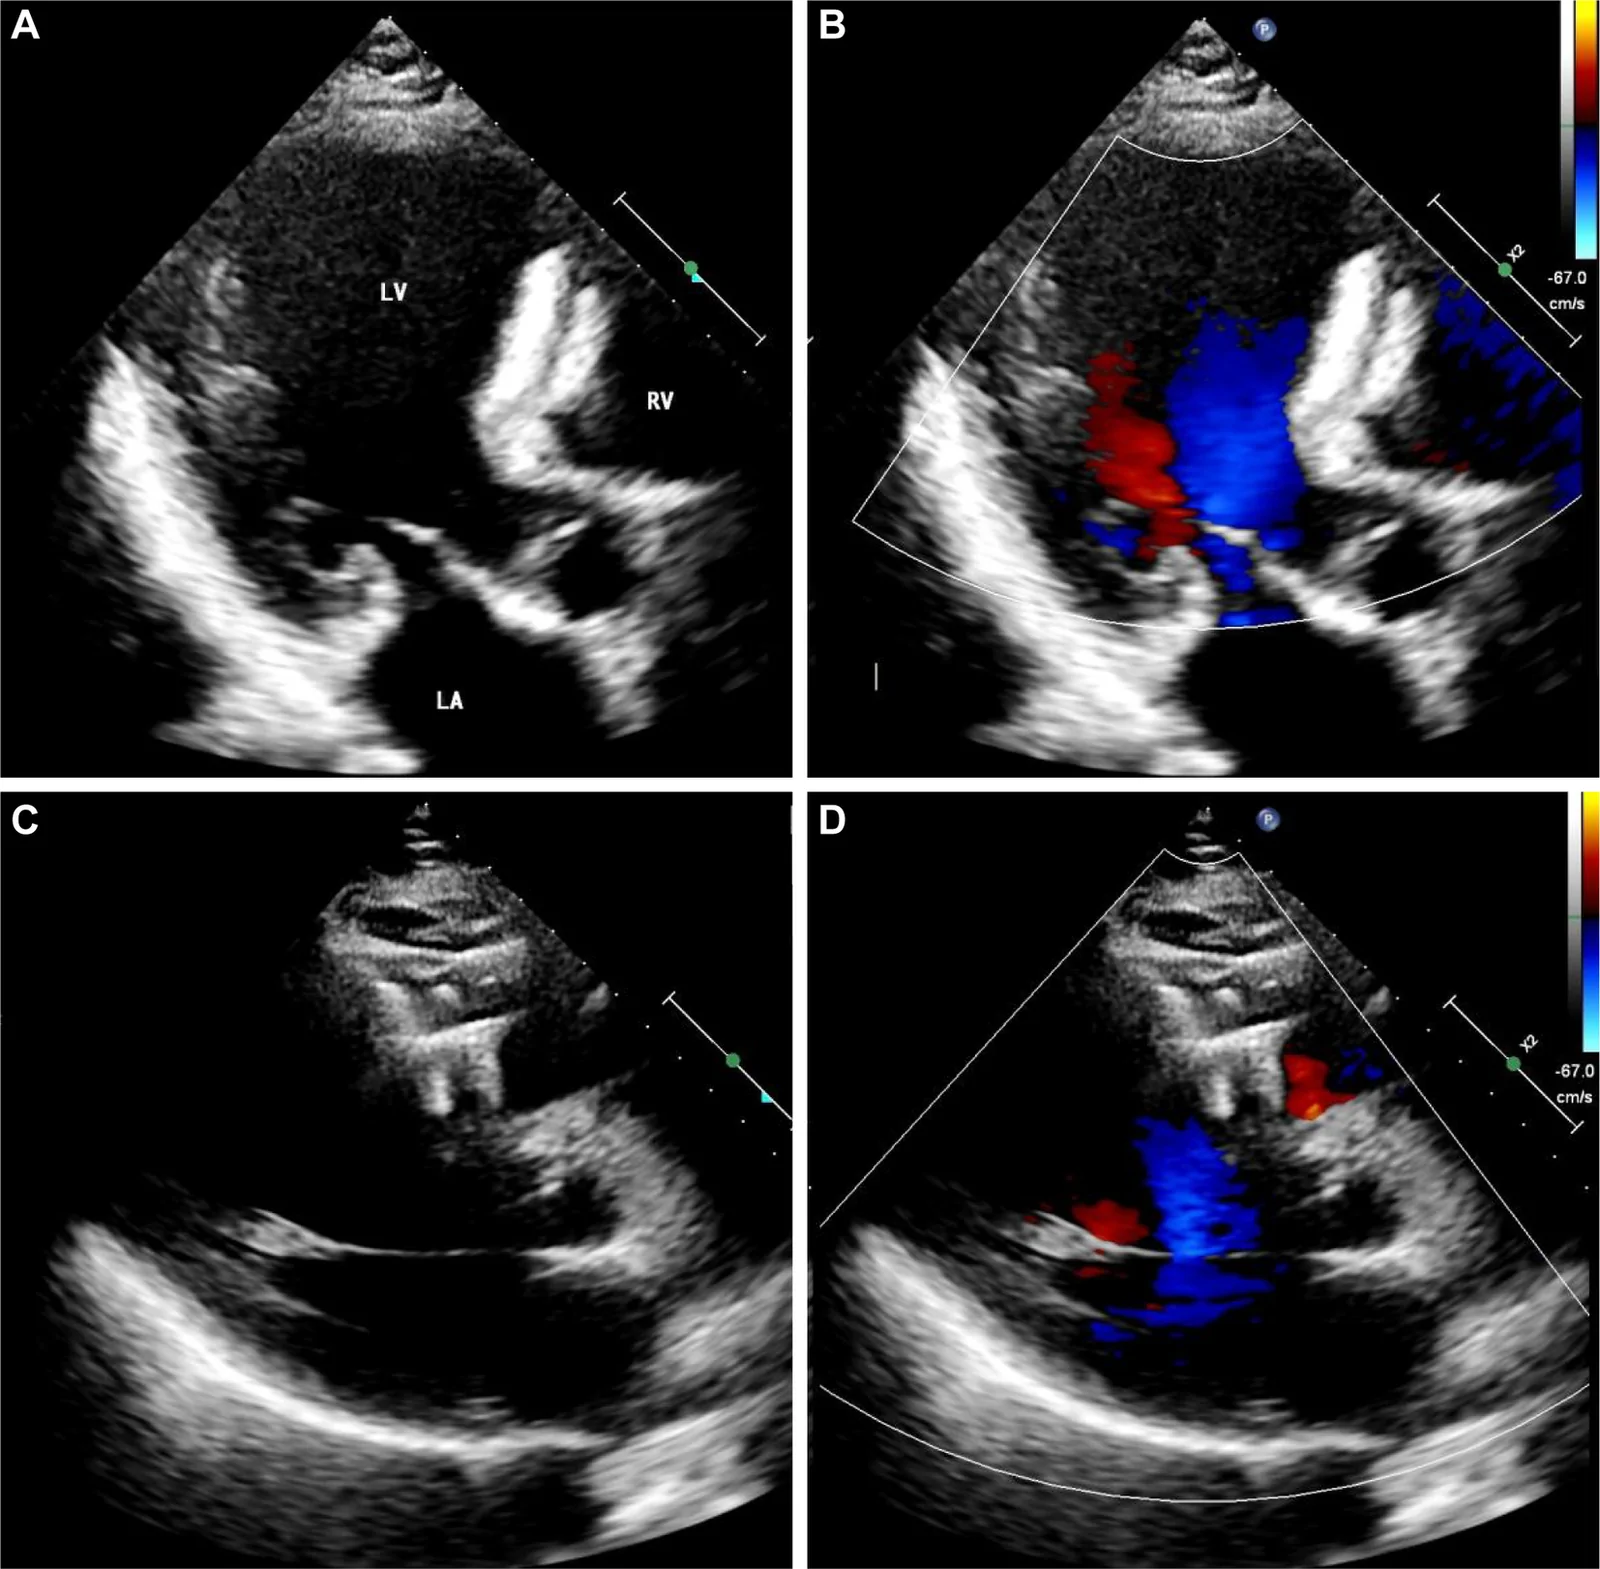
(Panels A to D) displays the transthoracic echocardiography (TTE) images of a patient at 6 months following implantation of the VSRD: (A) represents the apical four-chamber view image acquired via TTE; (B) is the color Doppler flow imaging (CDFI) image corresponding to panel A; (C) represents the parasternal long-axis view image acquired via TTE; (D) is the CDFI image corresponding to panel C. All images indicate that the occluder is accurately positioned and well-apposed, with no significant residual shunt detected.
